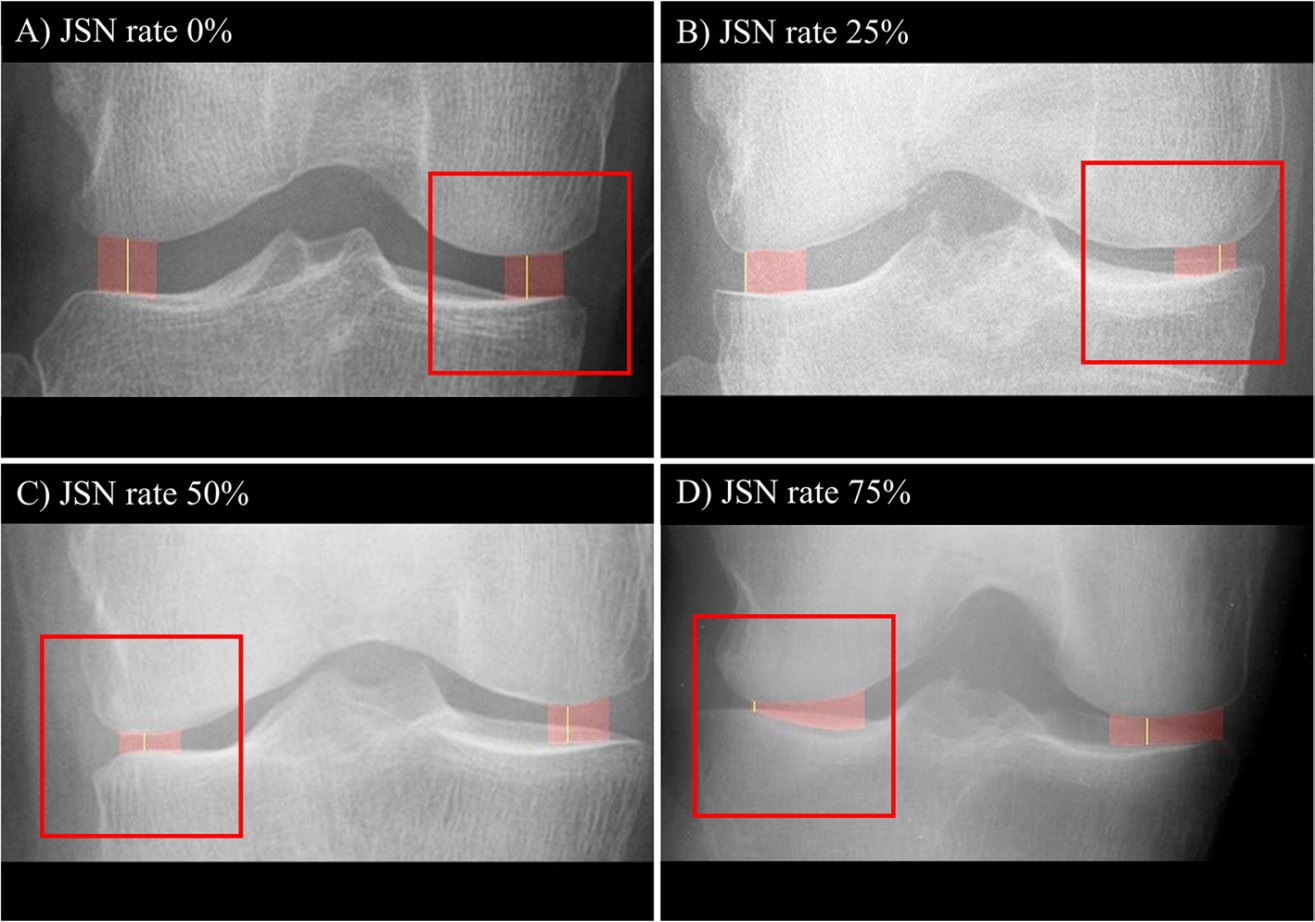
Example radiographs with JSN rates A) 0%, B) 25%, C) 50%, and D) 75%. Among medial and lateral joint space, the red square box is the area corresponding to each percentage
